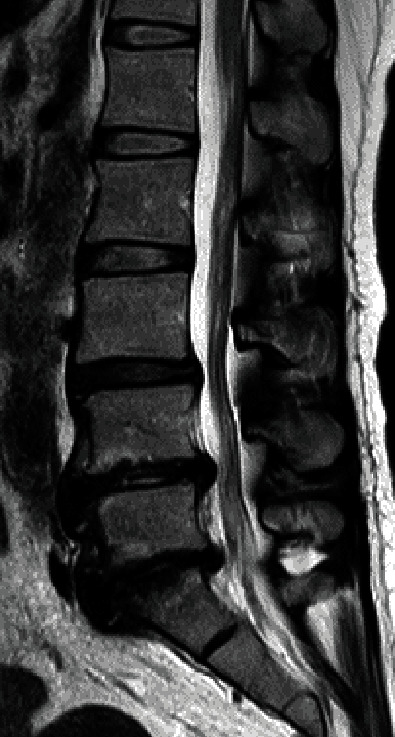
T2-weighted magnetic resonance imaging (MRI) of the lumbar spine of a 49-year-old woman with degenerative lumbar disease. Midsagittal section.
